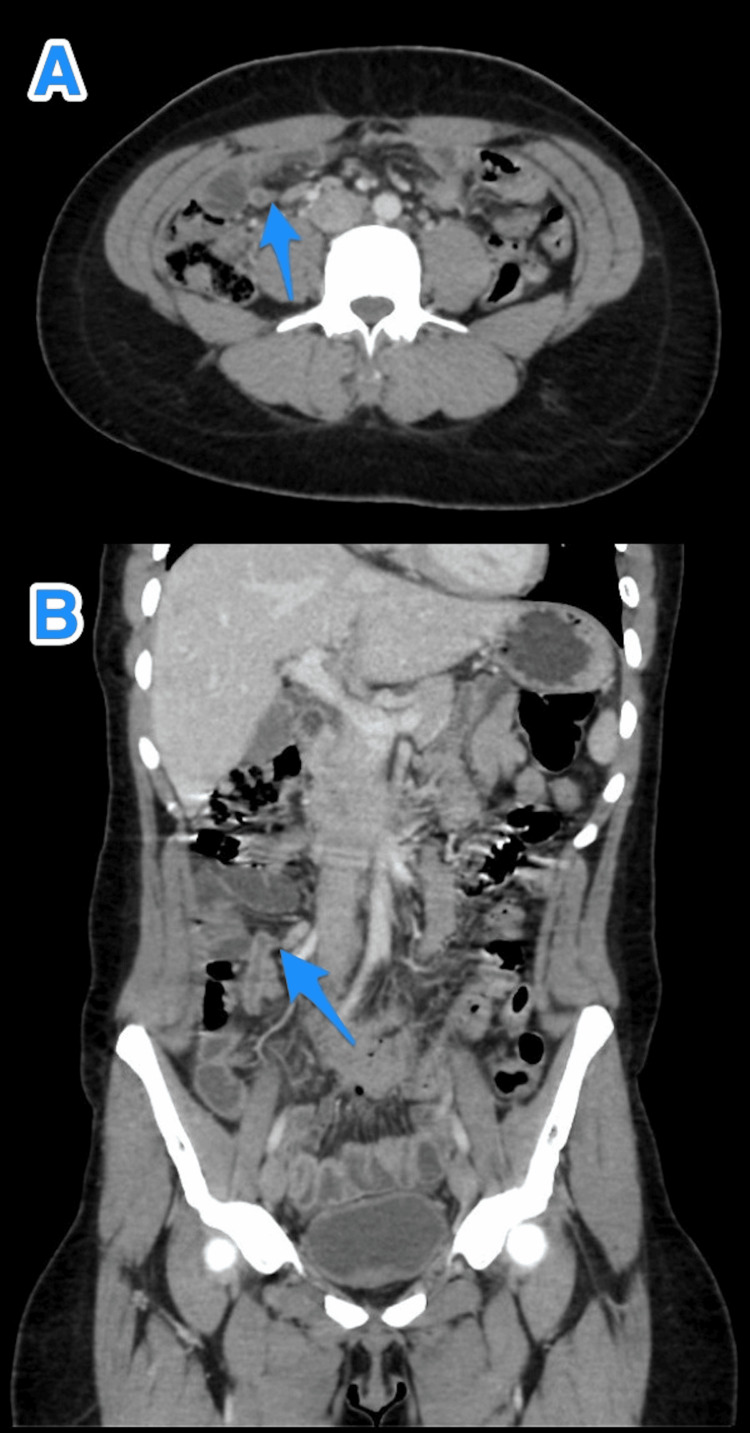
A multiview computed tomography abdominal scan (A and B) shows a small diverticulum close to the tip of an inflamed appendix (arrows).
